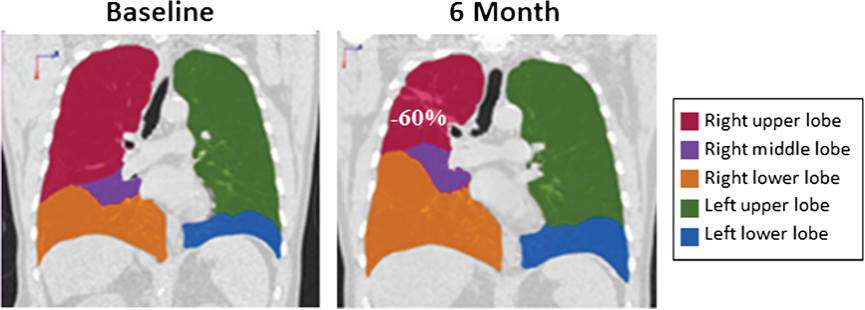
Radiographic image of lungs pre- and post-vapor ablation treatment. Radiographic image shows treatment of the right upper lobe resulting in targeted lung volume reduction with expansion of lower lobe following vapour ablation (VAPOR trial)
